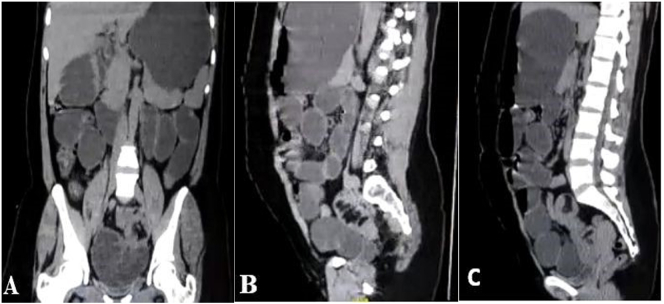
(A) Coronal section CT scan showing grossly dilated stomach and small bowel loops. (B) and (C) Sagittal sections showing bowel within bowel configuration, evident by presence of small bowel mesentery within bowel lumen.
